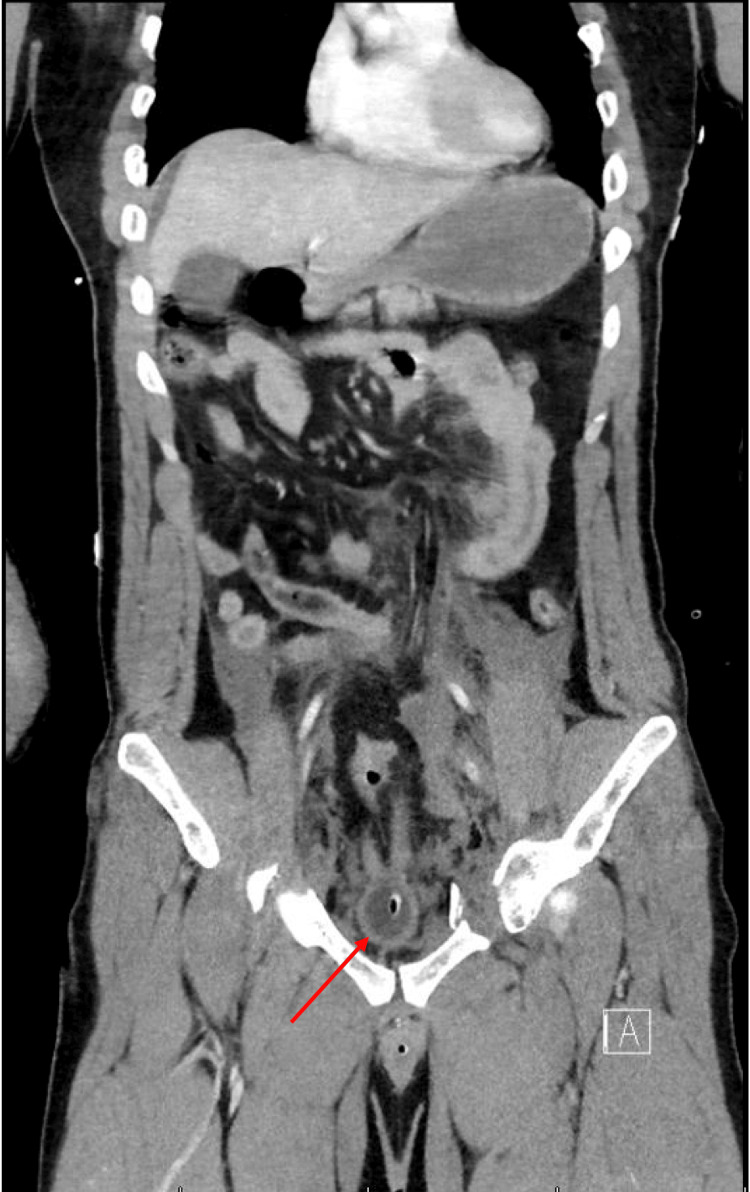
Abdominal CT scan highlighting the bladder. Coronal abdominal CT scan revealing an extraordinarily compressed bladder, which could have been the result of a potential bladder rupture, especially in the context of a pelvic crush injury or open-book pelvic fracture. The compression of the bladder suggests that it has been subjected to substantial external pressure, likely from displaced pelvic bones or direct traumatic forces. This degree of compression increases the risk of bladder wall rupture. Furthermore, the presence of free fluid in the retroperitoneal space on the CT scan supports the diagnosis of a ruptured bladder, as urine may leak into this space following a tear in the bladder wall. Note: The bladder is indicated by a red arrow.
